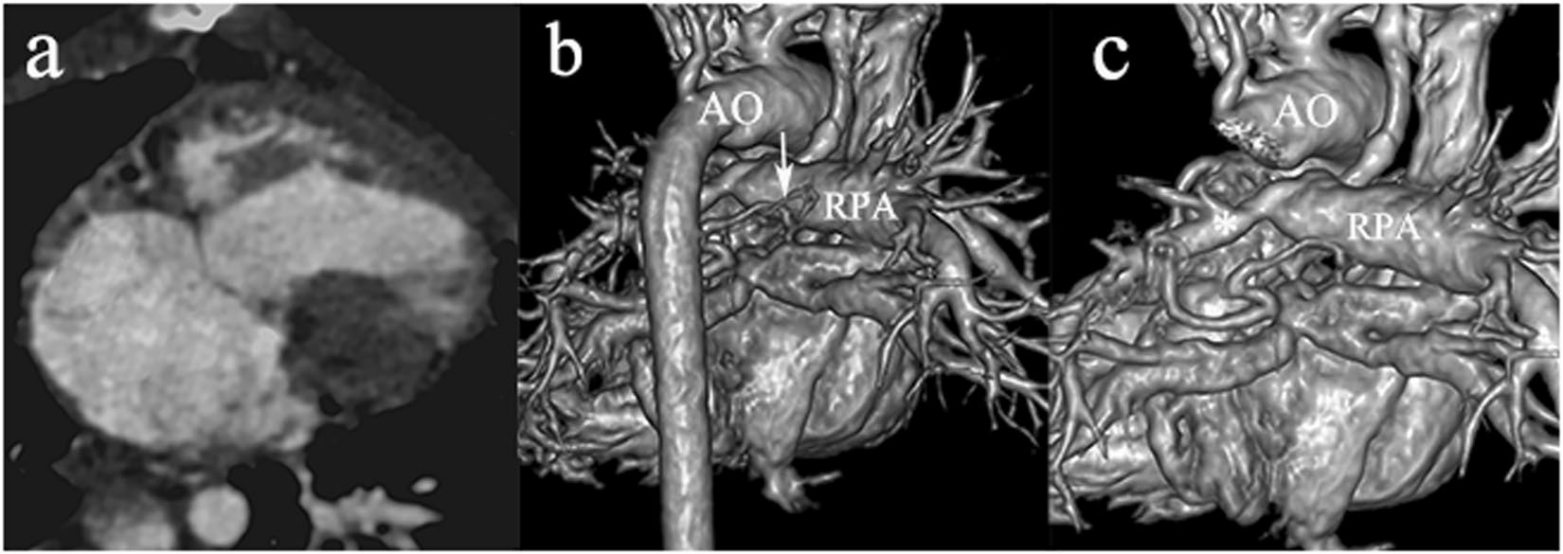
Accompanying arterial malformations detected by DSCT in a 9-year-old female with SV. (a) The transverse plane of univentricle of the patient. (b) The systemic-to-pulmonary collaterals detected by DSCT: The collaterals (arrow) arise between RPA and AO. (c) The PA distortion detected by DSCT: LPA (asterisk) is markedly hypoplastic. AO indicates aorta; DSCT, dual-source computed tomography; LPA, left pulmonary artery; RPA, right pulmonary artery; SV, single ventricle.
